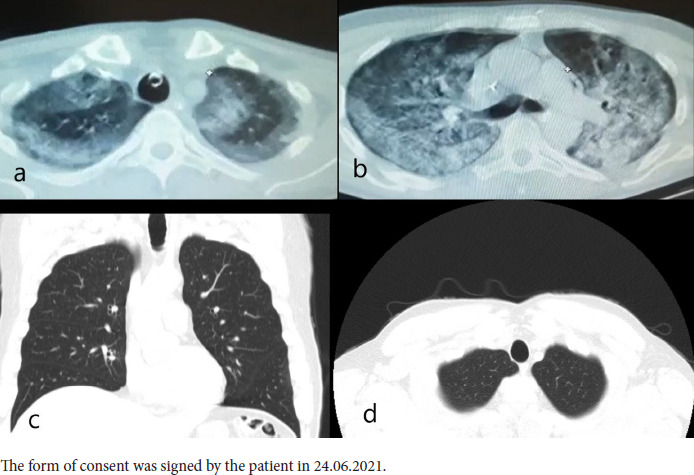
(a). The CT scan performed at the public hospital showed consolidation and GGO areas corresponding anatomically to the same location with apical reticular and bullous changes at the bilateral pulmonary apex. (b). Generalized consolidation and GGO were observed in the axial CT image from carina level. (c). Coronal and (d). Axial CT images before infection with COVID-19 showed that the pulmonary apices were clear.
